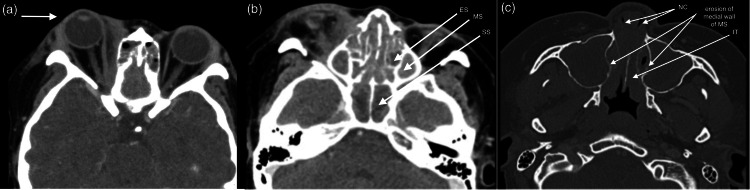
CT scan of the brain and orbit showing right eye proptosis (arrow) a: complete soft tissue opacification was seen in all the paranasal sinuses; b: the medial wall of both maxillary sinuses eroded, and soft tissue thickening was seen in both nasal cavities; c: bilateral middle and inferior nasal turbinate hypertrophied ES: ethmoid sinus; MS: maxillary sinus; SS: sphenoid sinus; NC: nasal cavity; IT: inferior turbinate
